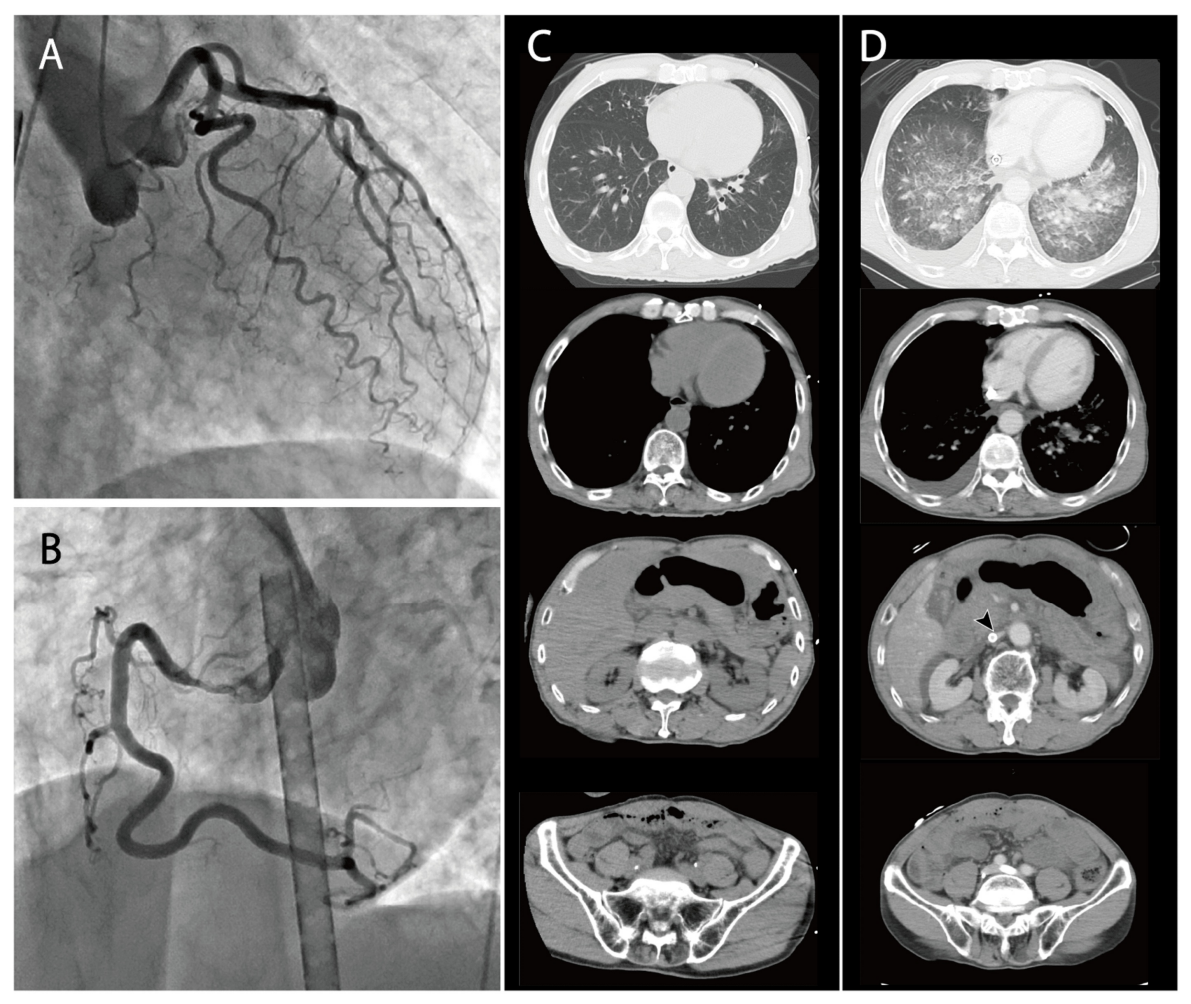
Diagnostic Imaging Results (A, B) Coronary angiogram of the left coronary artery (A) and the right coronary artery (B) revealed no significant stenosis. (C) Computed tomography (CT) obtained at the referring hospital did not show any evidence of chronic heart disease or infection foci. (D) Post-extracorporeal membrane oxygenation (ECMO) initiation contrast-enhanced CT scan demonstrated thickening of the interlobular septa (arrowhead), findings consistent with alveolar pulmonary edema, as well as the presence of pleural and ascitic fluid. The ECMO cannula was inserted into the inferior vena cava, which was collapsed.
